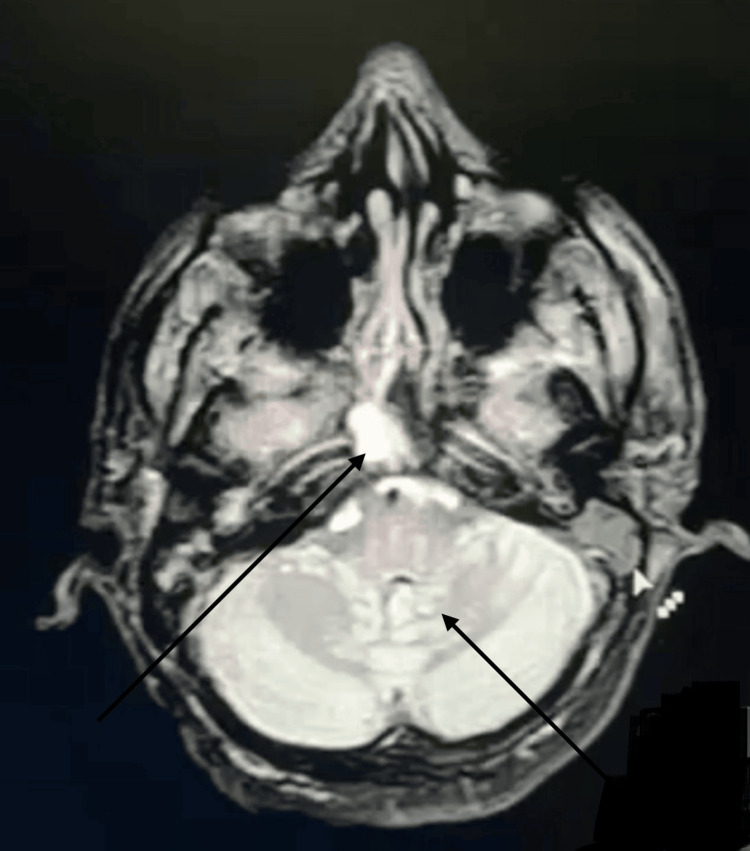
Follow-up axial T2-weighted MRI of the brain at eight weeks demonstrating residual signal changes in the central pons with reduced hyperintensity compared to the initial scan, consistent with resolving central pontine myelinolysis and residual gliosis. No gadolinium contrast was administered.
